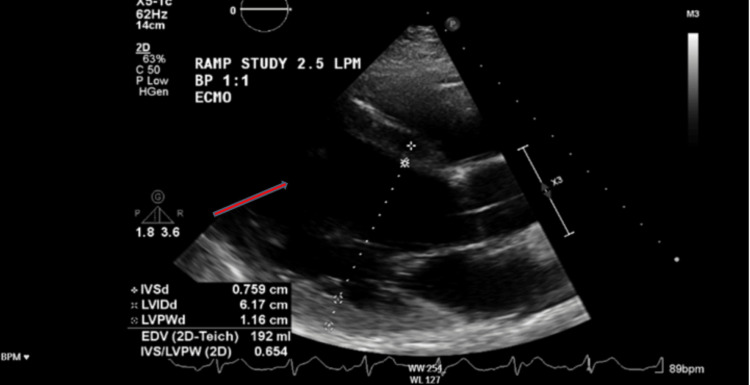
TTE parasternal long-axis view showing a severely dilated left ventricle TTE: transthoracic echocardiogram
